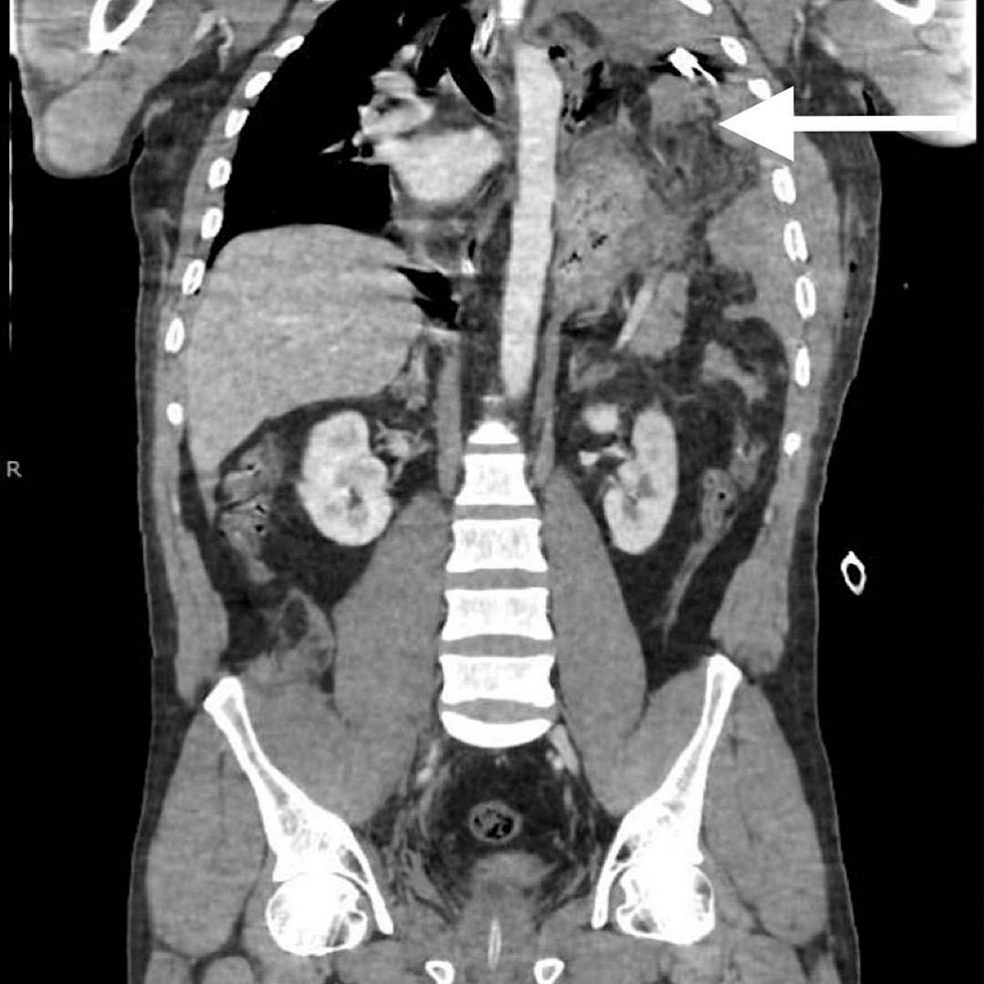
CT scan (coronal view) showing abdominal visceral herniation (arrow) into the left hemithorax.
